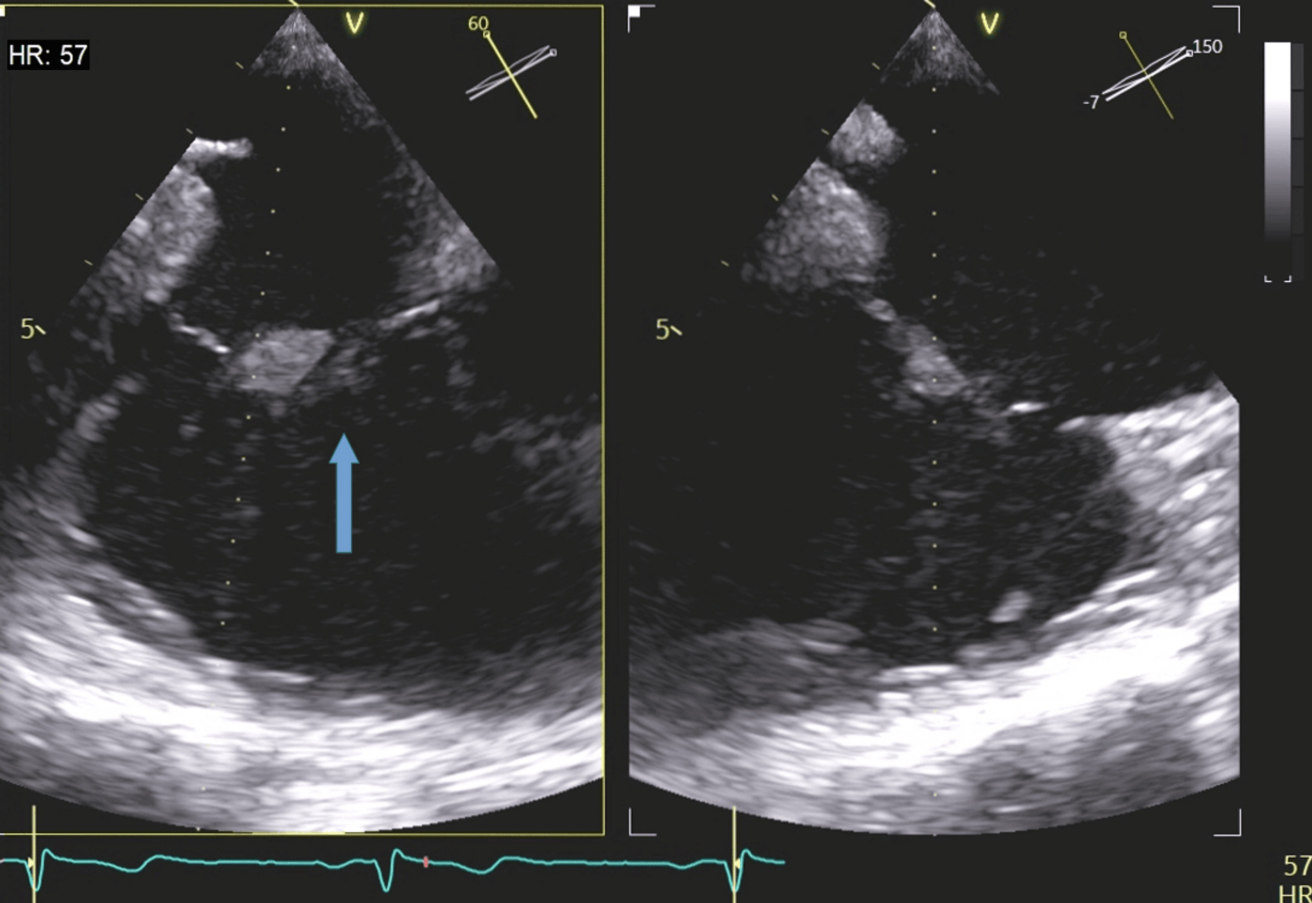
Transesophageal echocardiogram (TE) shows endocarditis of the native tricuspid valve
